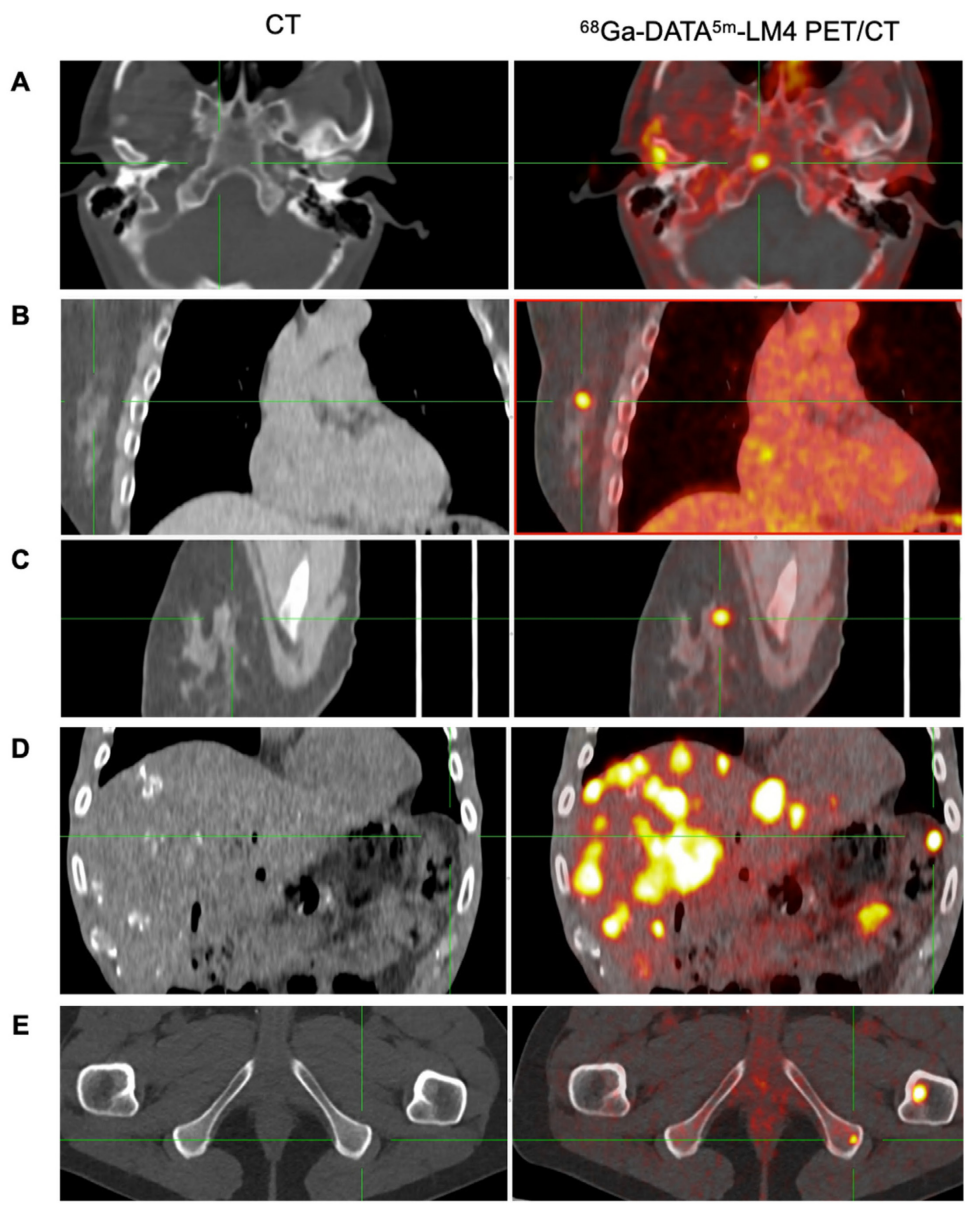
Representative images of ^68^Ga-DATA^5m^-LM4 PET/CT demonstrating the detection of tumor metastases that are undetectable on conventional CT, located in: skull base (A), right breast (B and C), peritoneum (D), and ischial bone (E).
